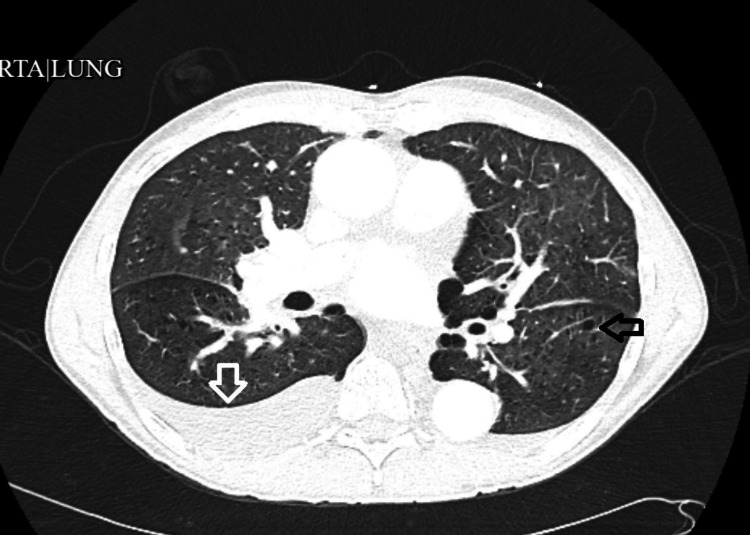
Computed tomographic angiography of the chest showing moderate emphysema (black arrow) and pleural effusion (white arrow).
